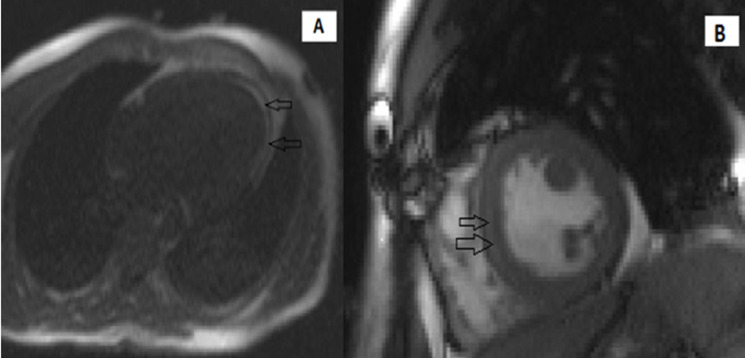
MR imaging findings: (A) horizontal long axis true-FISP performed after gadolinium infusion demonstrated a subepicardial enhancement (arrows); (B) short-axis showing delayed-enhancement imaging (arrows). True-FISP = True Fat Imaging with steady-state precession
